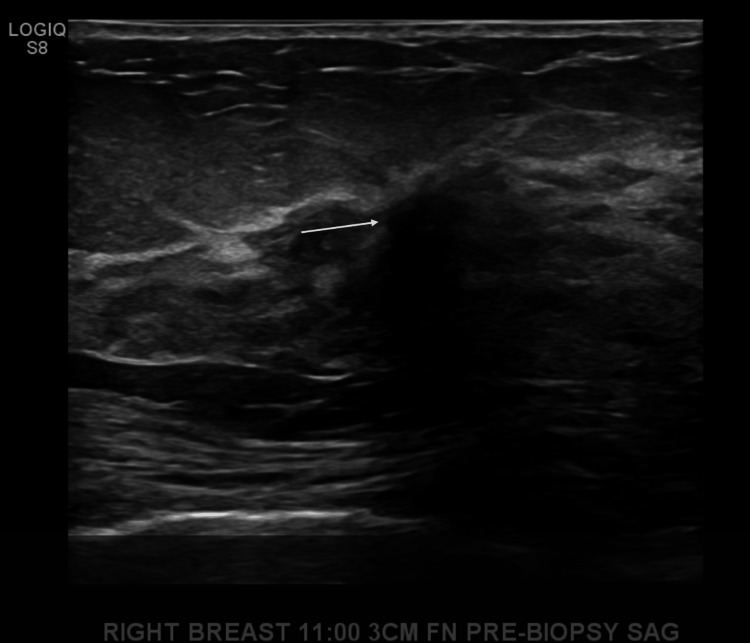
Ultrasound of the right breast from case one. Shows a hyperechoic focus with ill-defined borders measuring 6 x 8 x 6 mm in the 11 o’clock position, 3 cm from the nipple.
